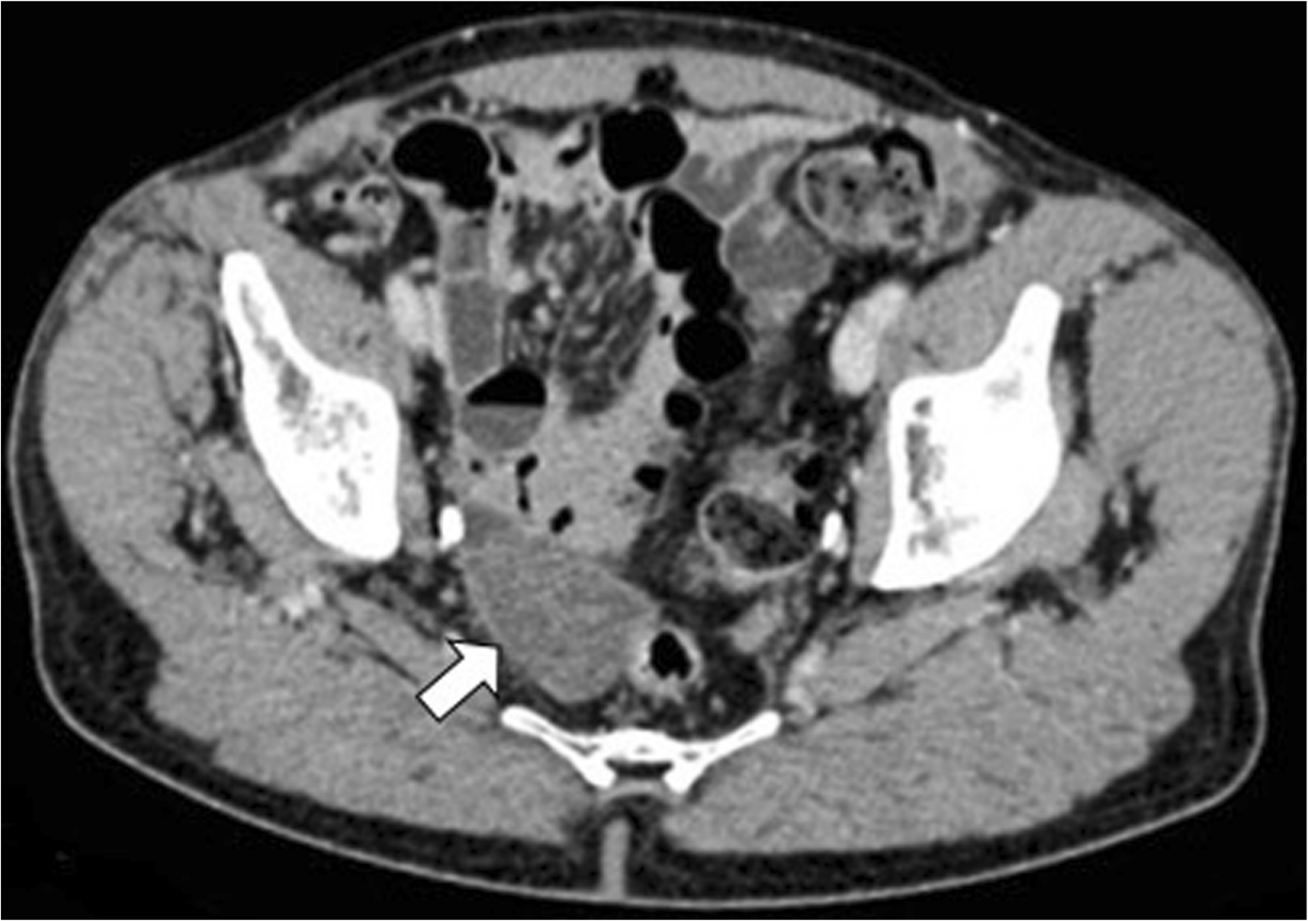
A 70-year-old man with echinococcosis. Axial CT image on portal venous phase demonstrates a pararectal homogeneous cystic lesion (arrow), showing internal septa and partial wall calcification, in a patient with hepatic hydatid cysts (not shown in this image)
